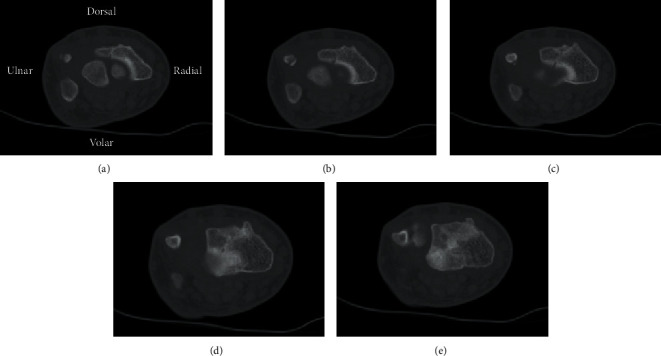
Sequential axial CT views (proximal to distal) demonstrating intra-articular extent of the fracture through the dorsal rim of the radial physis. (d) Shows the radial epiphysis fully in view, revealing the most volar extent of the fracture line dorsal to the volar ulnar rim.
